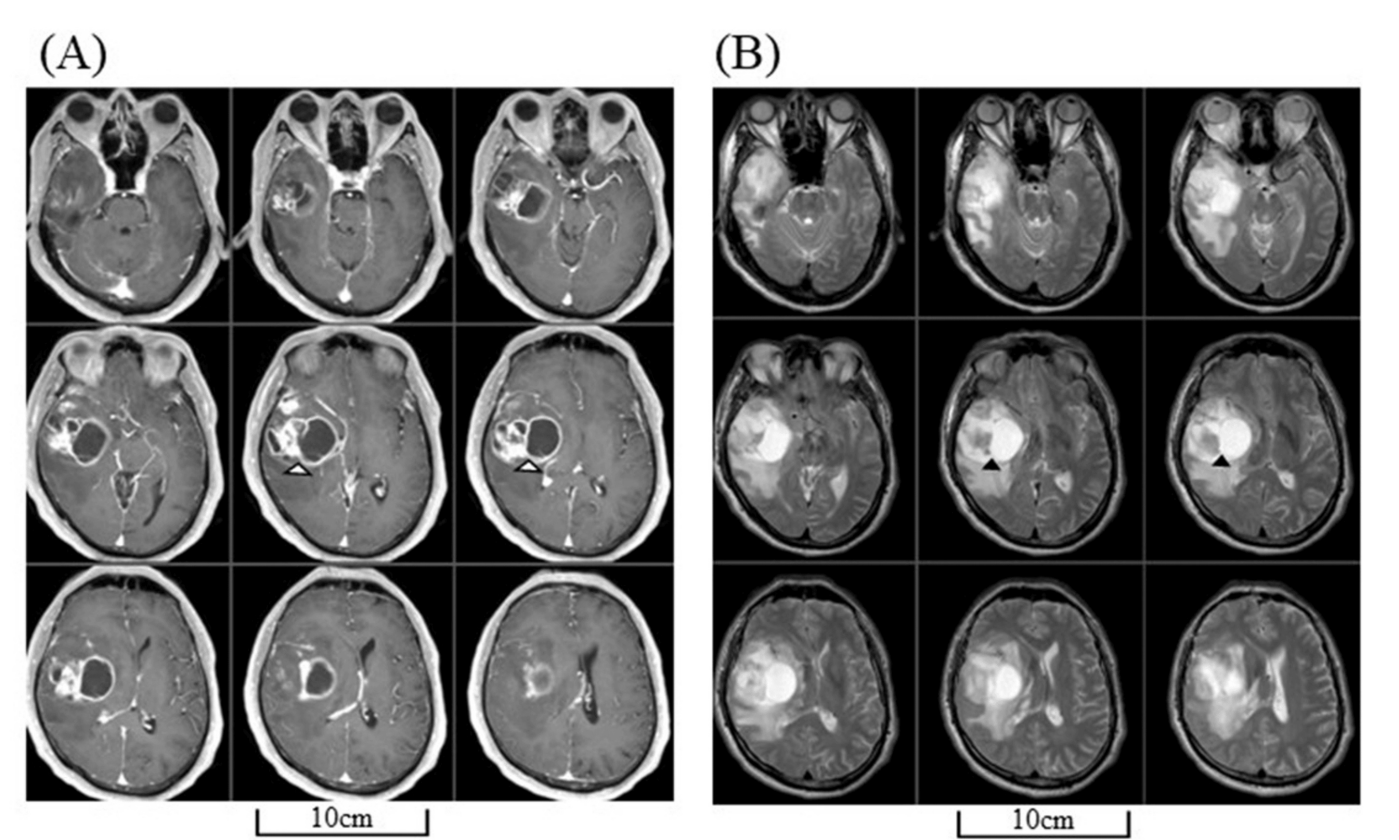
MRIs before surgery Head MRIs before surgery revealed a single mass lesion (50 mm in maximum diameter) located in the right temporal lobe (arrowheads). Post-enhanced T1-weighted images (A) and pre-enhanced T2-weighted images (B). MRI: magnetic resonance imaging
